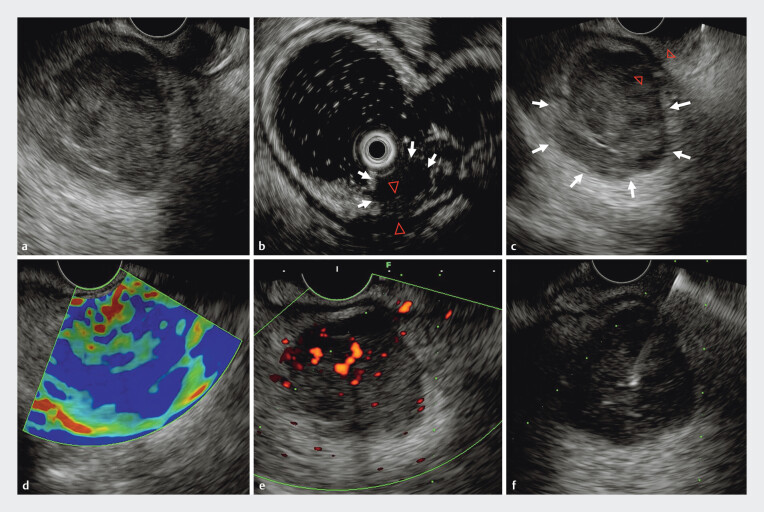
Endoscopic ultrasound (EUS) images.
